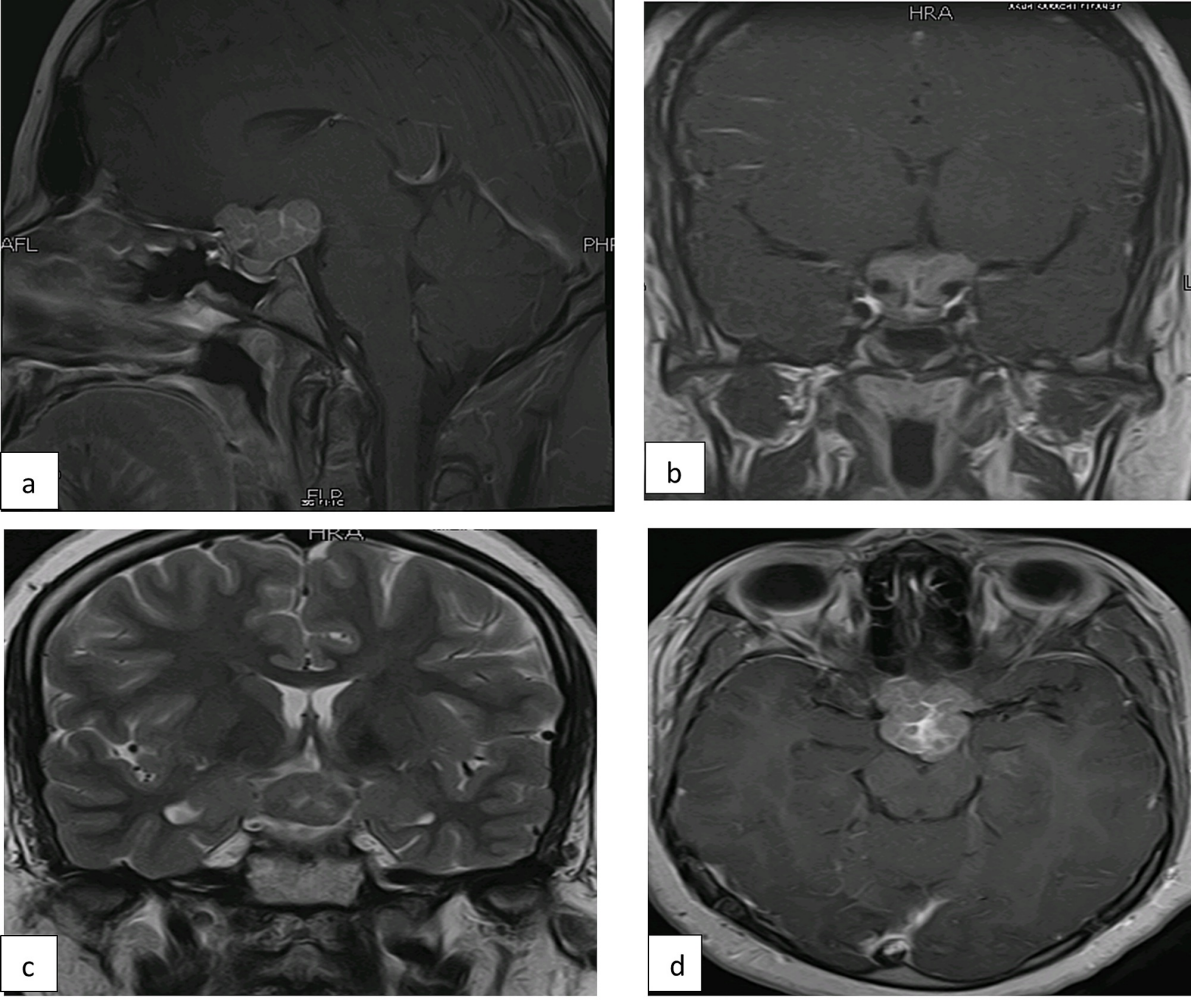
Follow-up MRI pituitary three months after surgery. It shows T1 postcontrast (a) sagittal, (b) coronal, (c) T2 coronal, and (d) axial images highlighting a heterogenous sellar mass with suprasellar extension with mild decrease in bulk overall. It is inseparable from the pituitary stalk and is displacing the optic chiasma superiorly.
